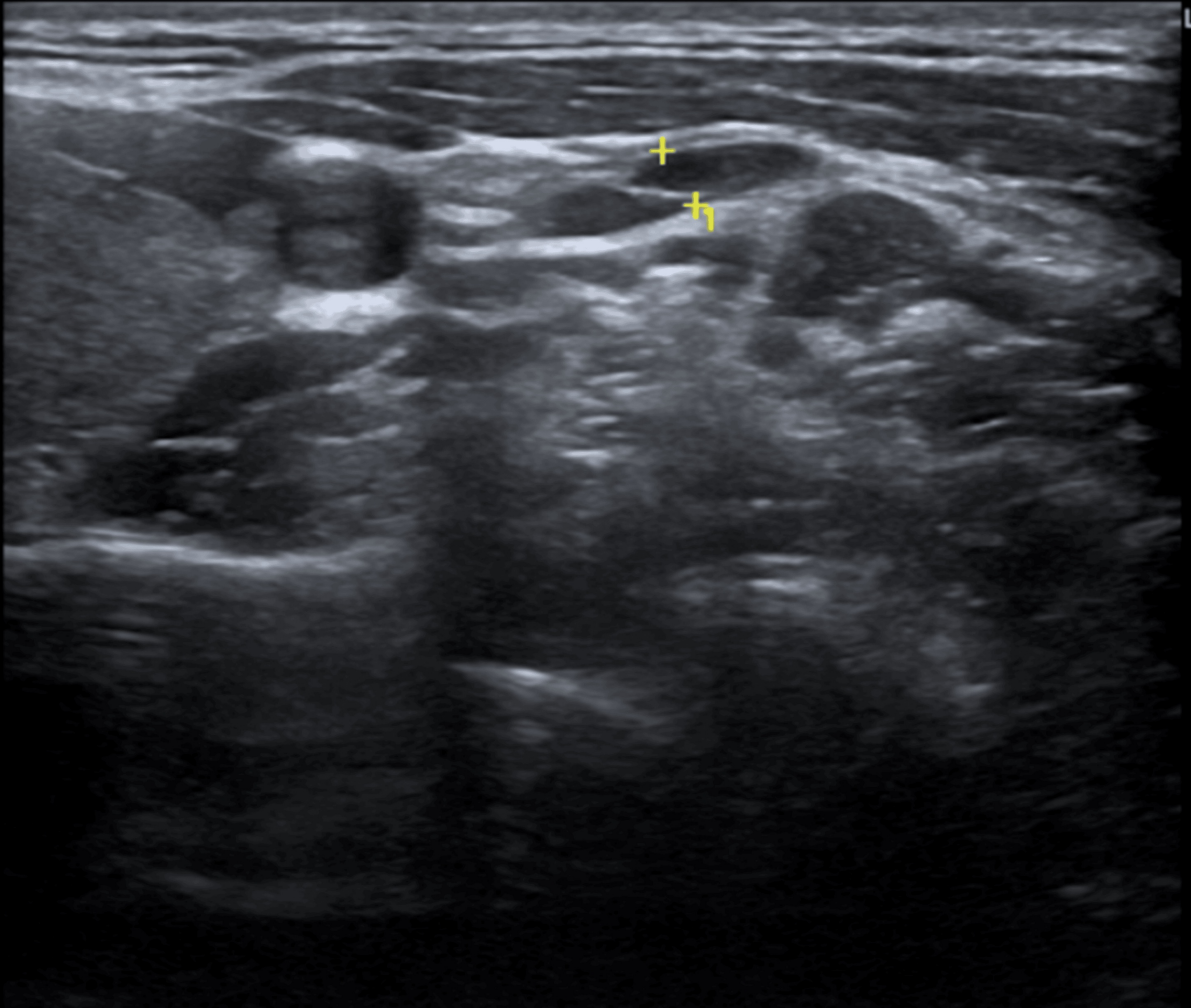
Thyroid ultrasound imaging
